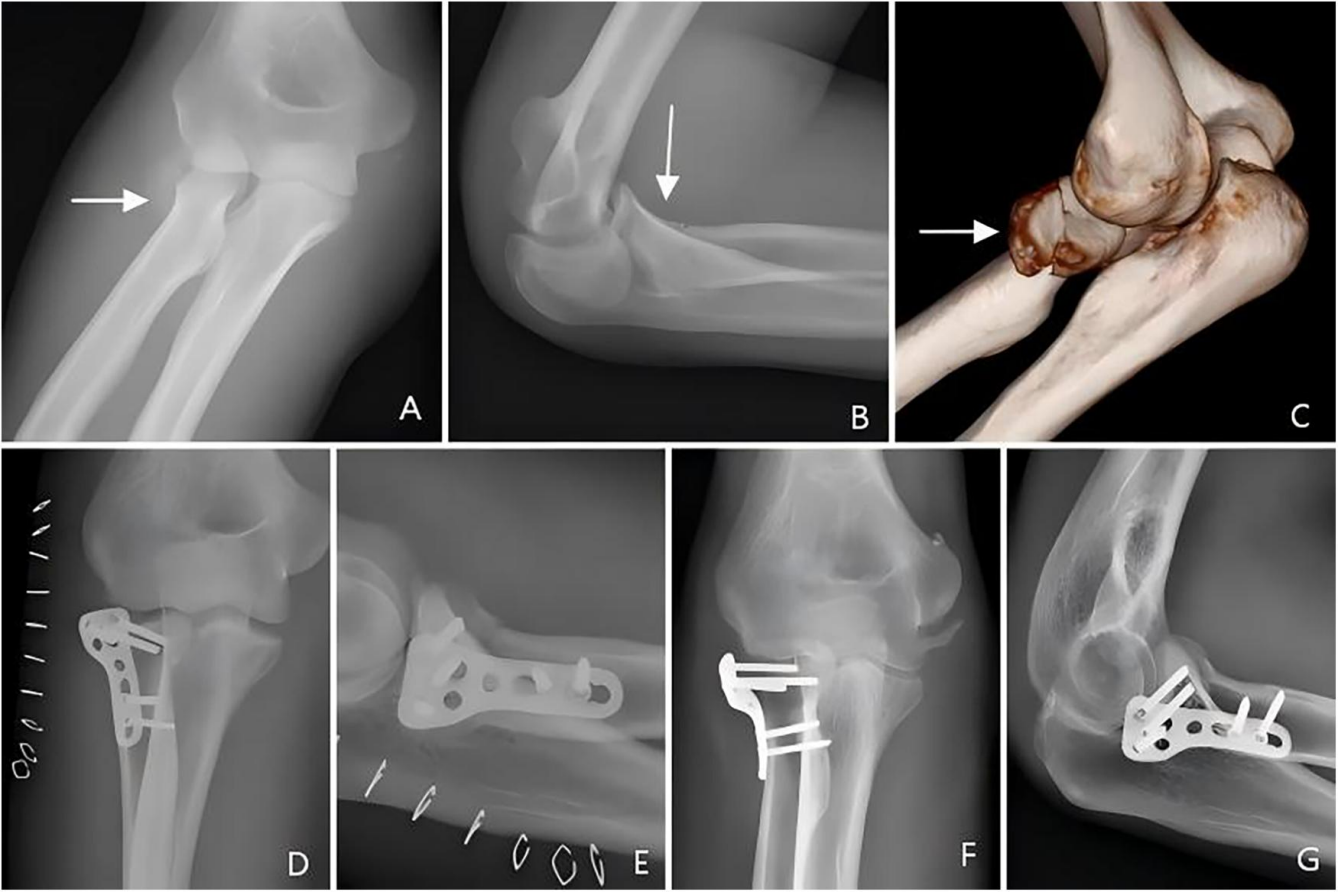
Radial head locking plate fixation: (A–C) Pre-operative AP/lateral views and 3D CT reconstruction showing radial head neck fracture with two fragments; (D,E) 1-day post-operative radiographs; (F,G) 15-month follow-up radiographs confirming fracture union.
